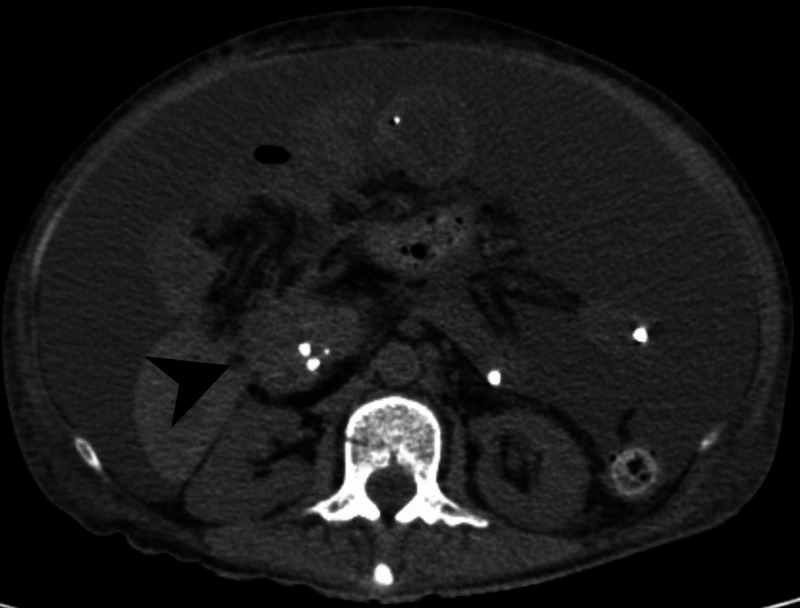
Abdominal Computed Tomography Black arrow highlights cephalic pancreatic mass
